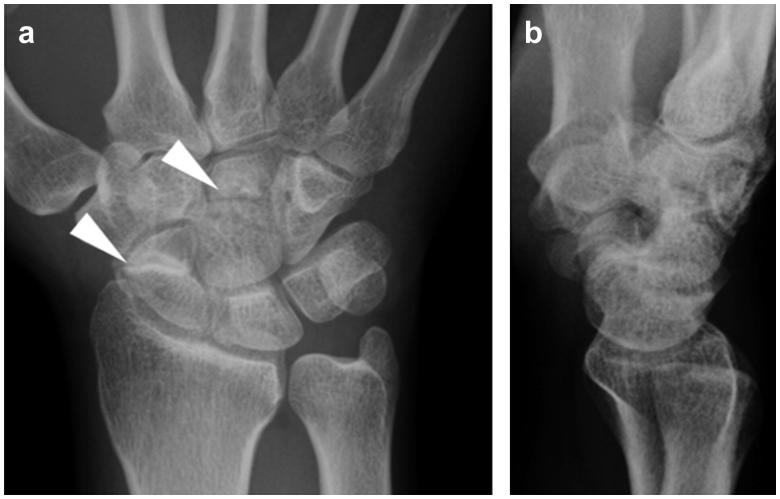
Radiographic assessment of the hand at our hospital. (a) Posteroanterior and (b) lateral plain radiographs showing scaphoid non-union and a radiolucent line in the central area of the capitate.
